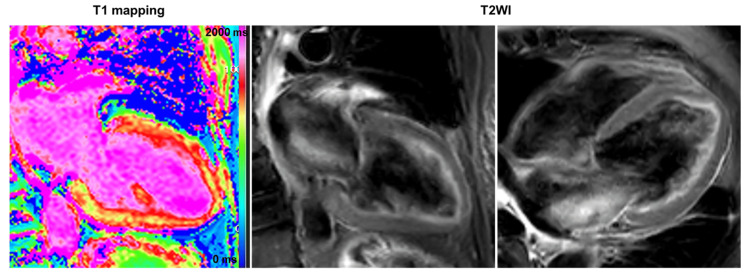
Cardiac magnetic resonance imaging (CMRI) on day 12 The CMRI indicated diffuse LV mild hypokinesis and prolonged native T1 (1365 ms; reference level 1314 ± 29 ms). Moreover, T2 in CMRI showed no obvious high-signal areas within the LV myocardium, and global T2 was 51 ms (reference level 46 ± 4 ms) with no obvious prolongation. There was no evidence of myocardial edema at T2.
